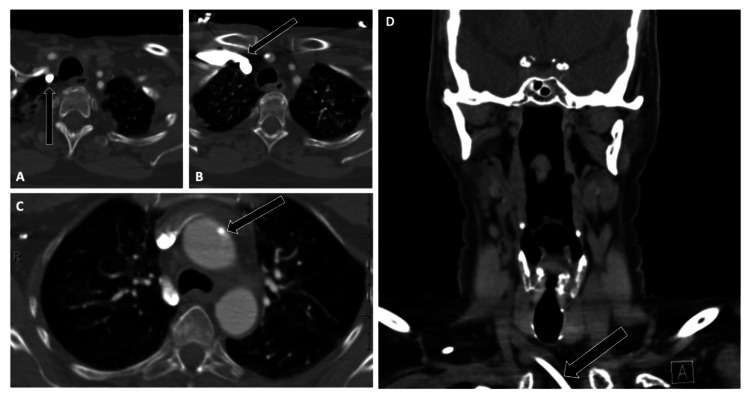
Arterial Malposition of a Central Venous Catheter on Chest CT Angiography Chest CT angiography demonstrating a chemotherapy catheter inadvertently placed within the arterial system.
